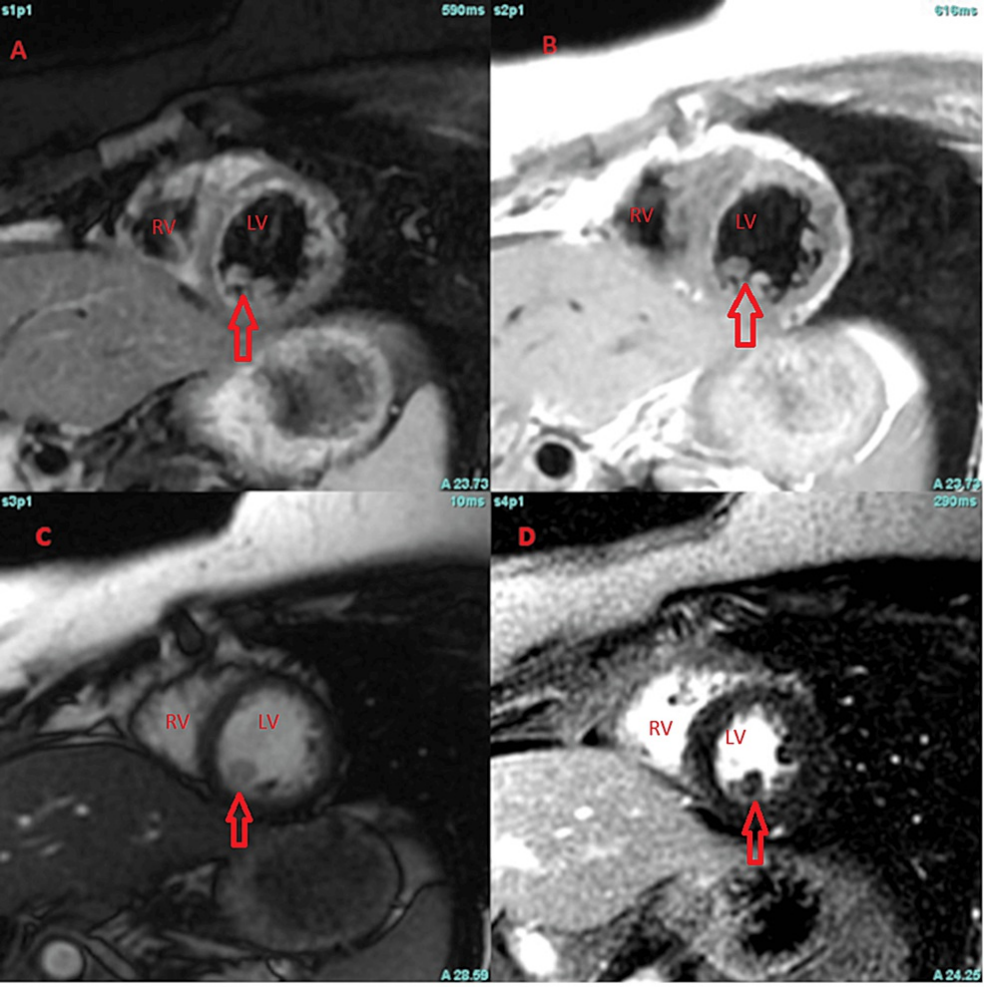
Cardiac MRI imaging A. Fat-suppressed black blood T2 weighted imaging (double Inversion recovery {DIR}, fast spin echo) in a short axis orientation demonstrating the mass to by isointense compared to the myocardium, which is more clearly demonstrated in long axis projections (see Figure 2). B. Black blood T1 weighted imaging (DIR, fast spin echo) in a short axis orientation demonstrating the mass to by isointense compared to the myocardium. C. Steady-state free precession (SSFP) imaging in a short axis orientation demonstrating the mass to by isointense compared to the myocardium. D. Delayed enhancement imaging demonstrating heterogeneous late gadolinium enhancement. LV: Left Ventricle. RV: Right Ventricle
